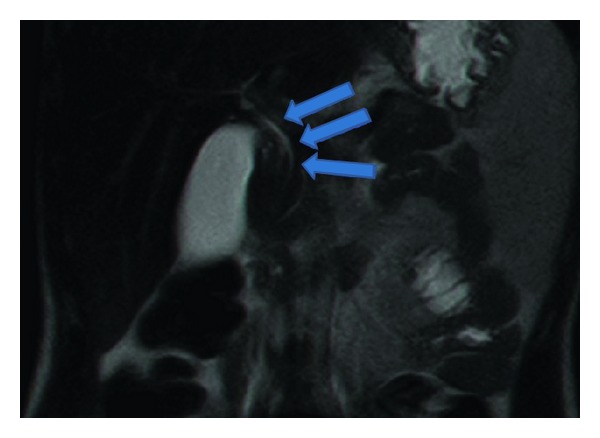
Coronal T2 MRI (as part of the MRCP STUDY) showing no dilatation and normal position of choledochus.
